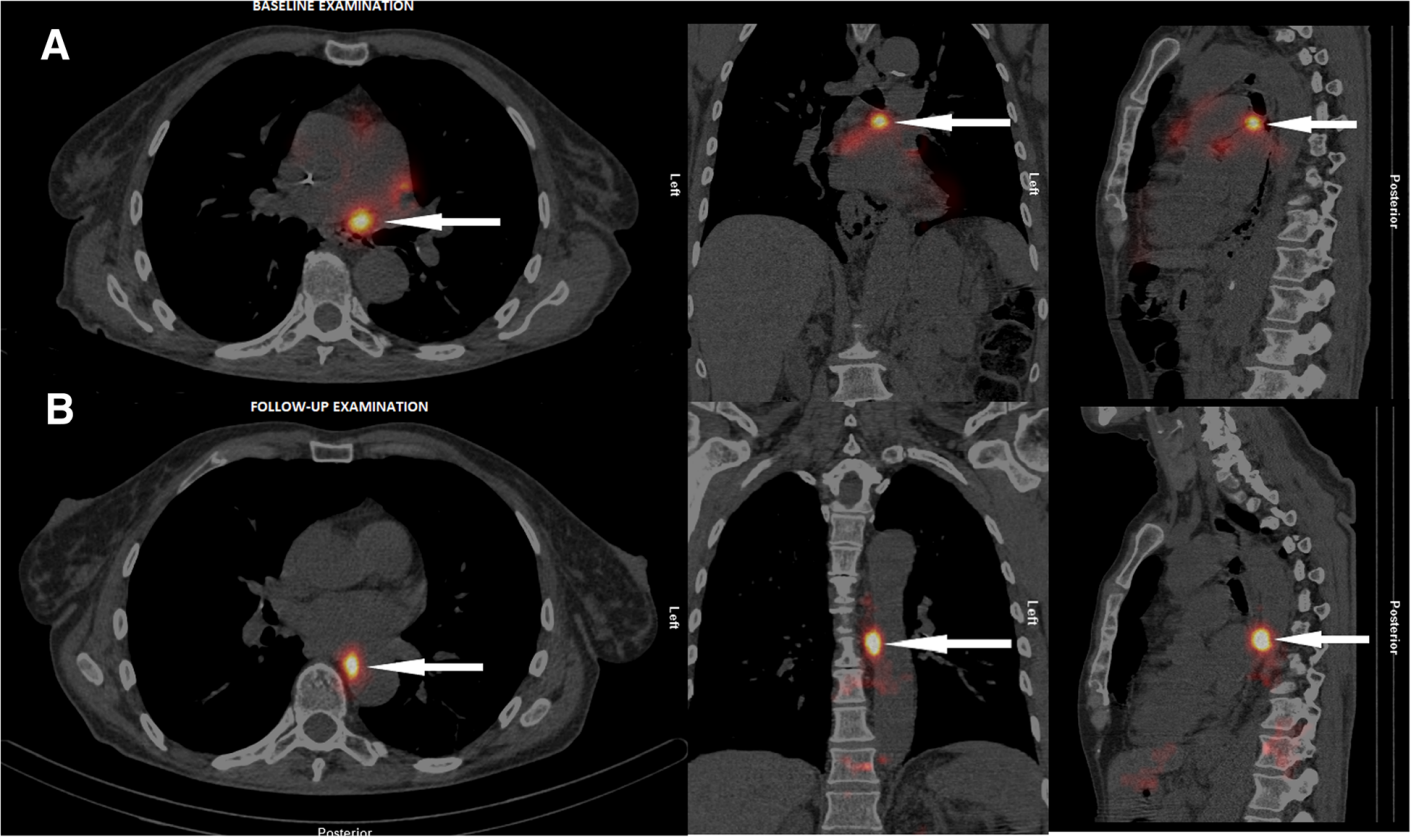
Hybrid SPECT/CT images of a 68-year-old female patient with a cT2 N0 M0 adenocarcinoma located in the cardia in axial, coronal and sagittal views from left to right in a) Baseline examination, b) Follow-up examination. White arrows indicate radio-colloid uptake. In the baseline examination a SLN was located in the 112 station. In the follow-up examination a SLN was instead located in the 109 L station
